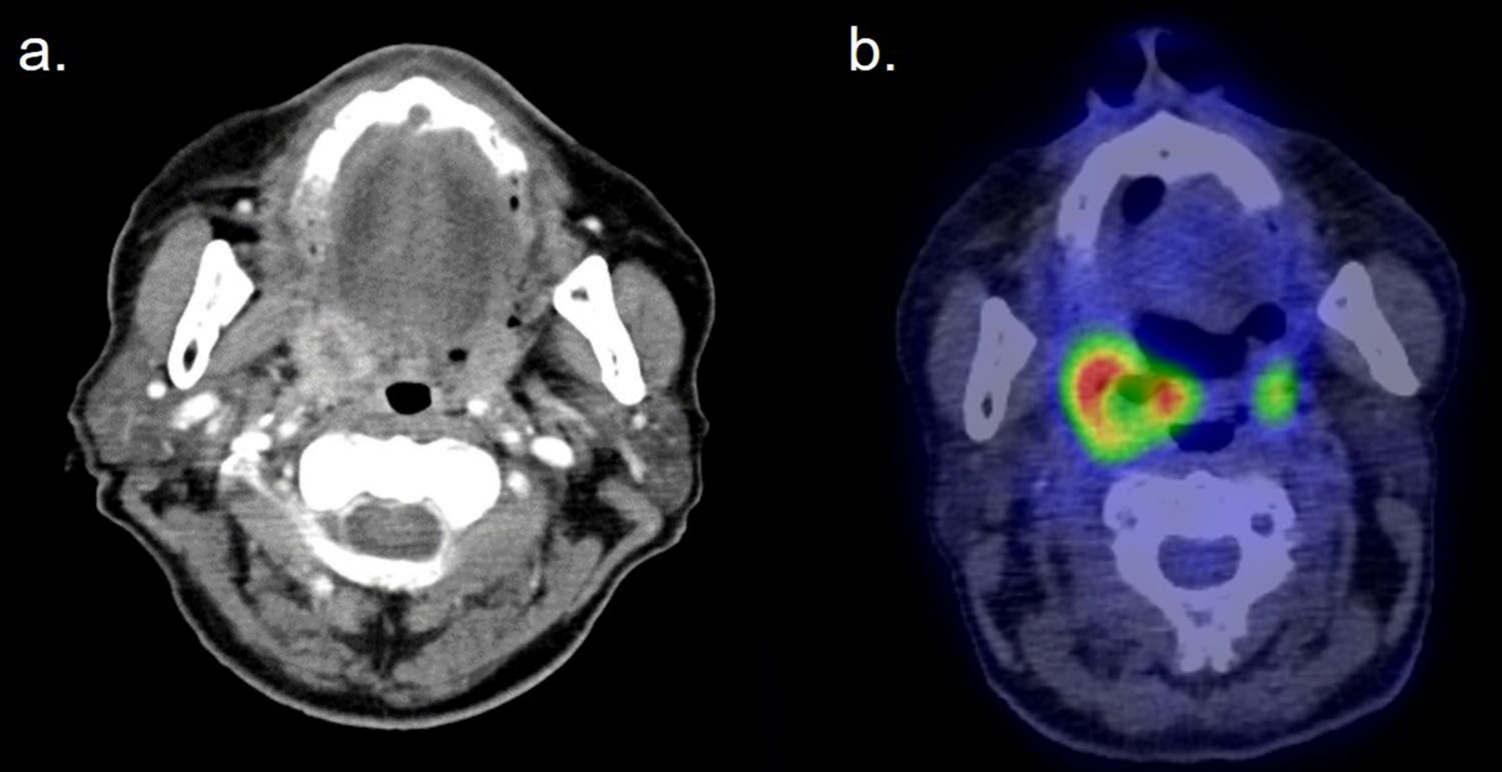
Patient with EBVMCU who had been receiving MTX for rheumatoid arthritis for 2 years. a Contrast-enhanced CT, b FDG–PET/CT fusion image
